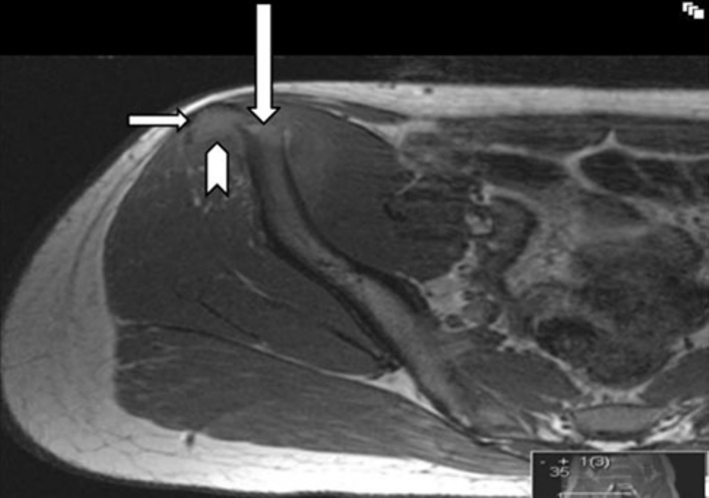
Axial T1-weighted image. Note the sharp anterior superior iliac spine fracture line (*long white arrow*) with retracted sartorius tendon margin (*short white arrow*). Also note that the signal intensity of haematoma is slightly hyperintense (as compared to skeletal muscle, indicated by the *chevron mark*), which is suggestive of a haemorrhagic product rather than a tumour mass
